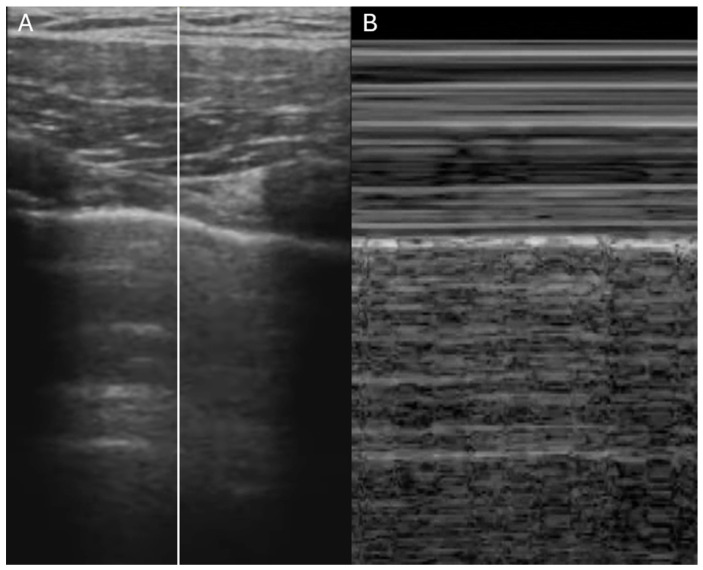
Lung ultrasound showing normal pleural sliding. (A) B-mode. (B) M-mode showing “seashore sign”.
